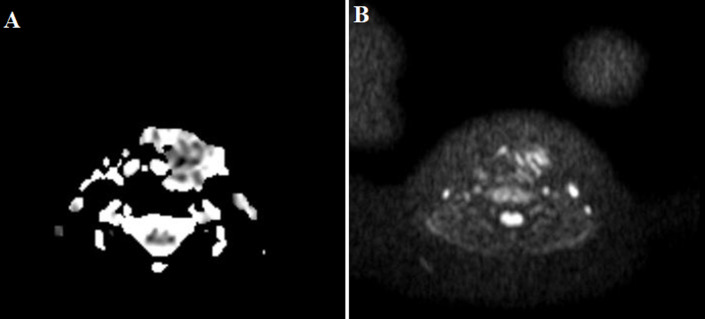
(A, B) apparent diffusion coefficient (ADC) map MRI and diffusion-weighted imaging (DWI); respectively to the configuration of the sinus track on the left is noticed bright signal on DWI and low signal on ADC map, confirming diffusion restriction because of pus content
